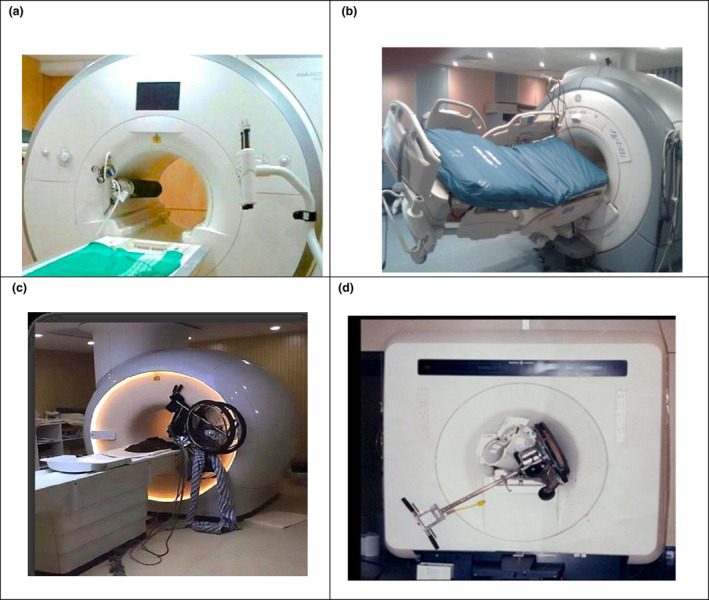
Examples of projectile accidents in the MRI environment: (A) oxygen cylinder, (B) stretcher bed, (C) wheelchair, (D) floor buffer. (Images courtesy of, and reprinted with permission from, Frank G. Shellock, Ph.D., www.MRIsafety.com).
